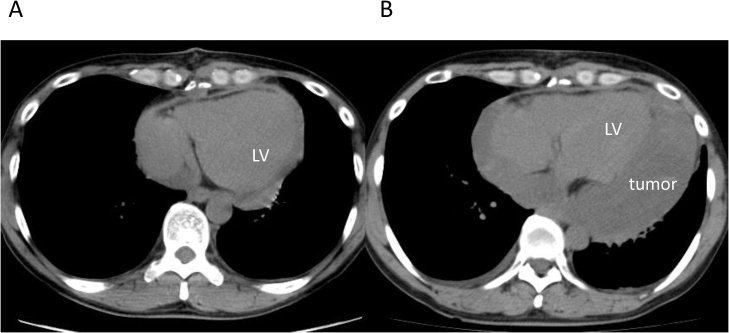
A. Computed tomography findings from postoperative day 10. The mass is almost gone. B. The findings of computed tomography 2.5 months after surgery. The regrown mass occupies the pericardial space and compresses the left ventricle (LV).
